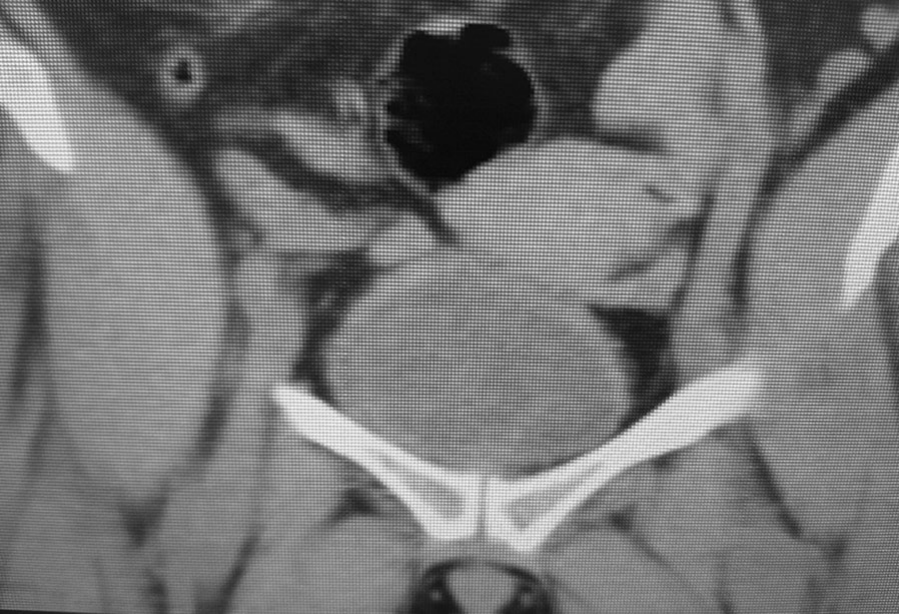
Noncontrasted CT with bladder mass
